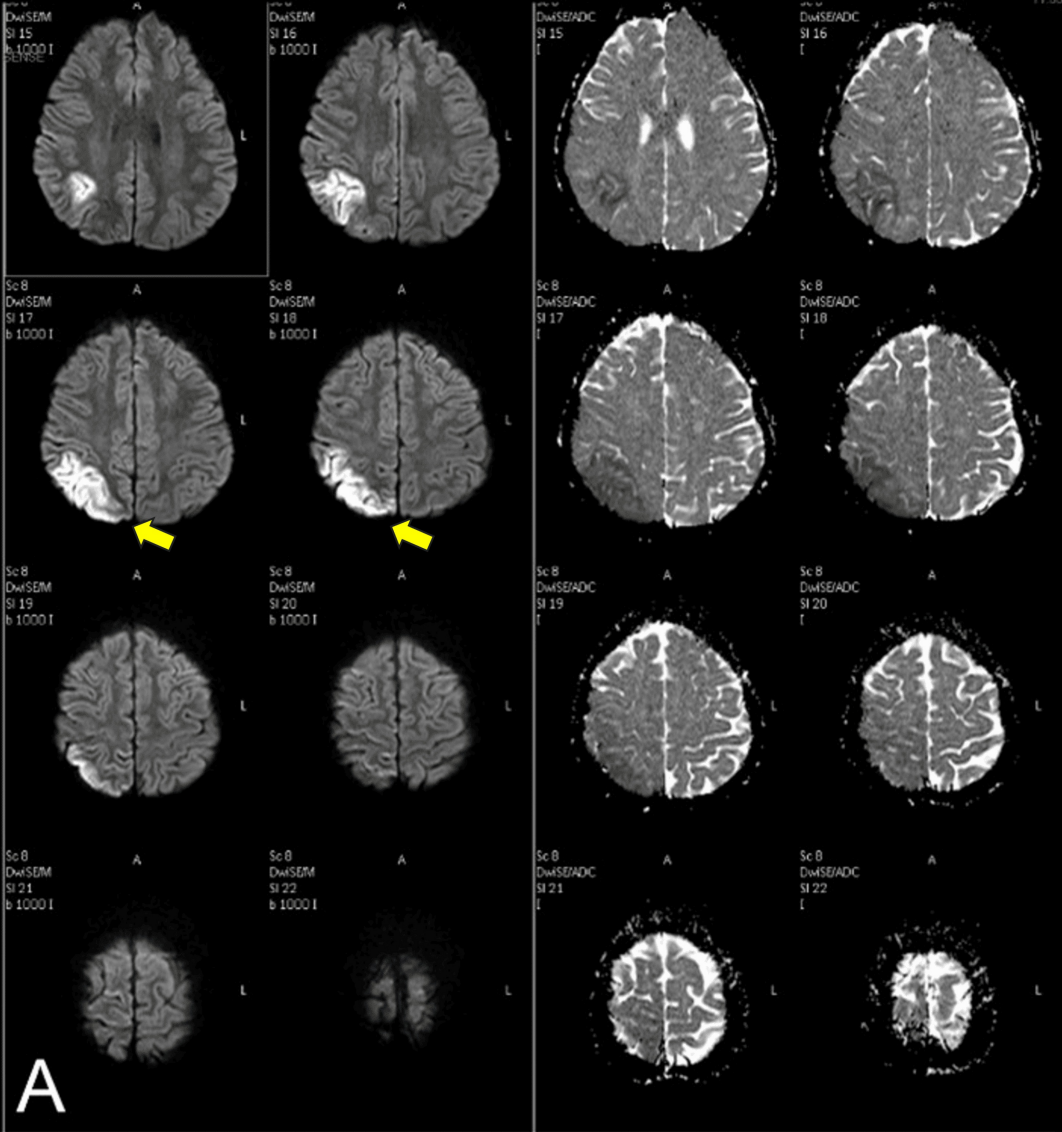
MRI examination of case 1. Diffusion-weighted imaging (DWI) and apparent diffusion coefficient (ADC) map show corresponding diffusion restriction in the right parietal cortical and subcortical region, consistent with an acute infarction.
